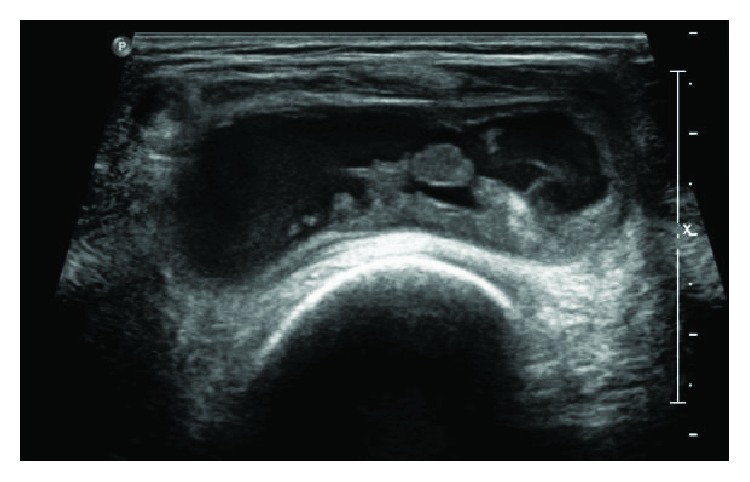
Ultrasounds of the left knee joint showing a joint effusion with villous hyperplasia of the synovial lining.
